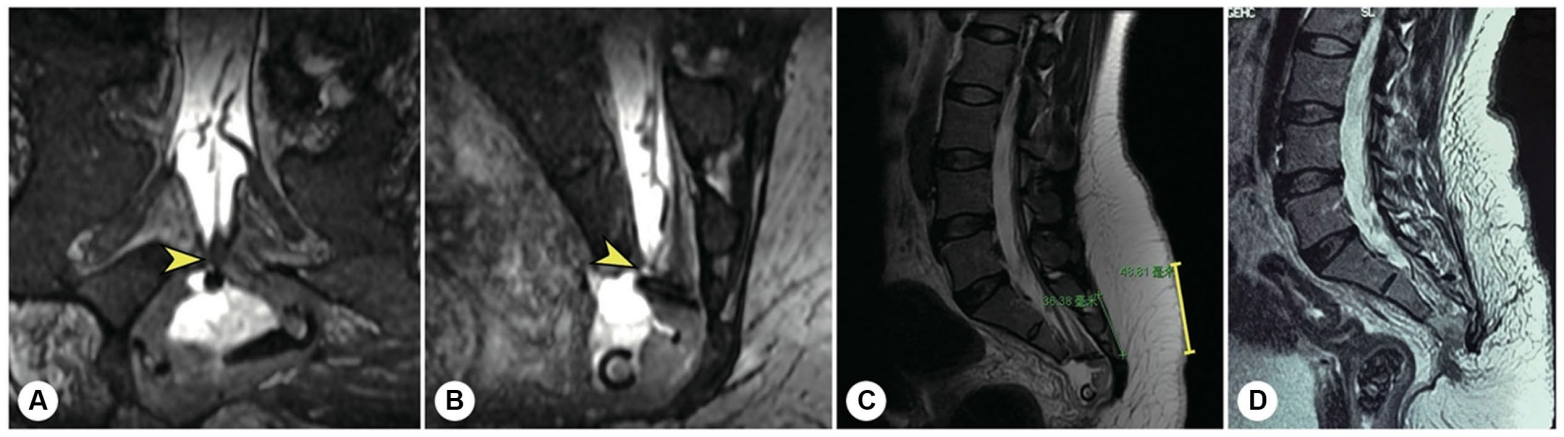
The other representative patient had both sacroanterior and sacral cysts. A 45 years-old female presenting with abdominal pain was found to have a pelvic mass on CT at the local hospital. The intrapelvic part of the cyst was resected under laparoscopy. (A) After referral to our institute, coronal reconstructed MR image showed a narrow-neck leakage orificium (yellow arrowhead) between the end of the dural sac and the sacral canal cyst, and the filum terminale was thickened. (B) Sagittal reconstructed MR image showing the leakage orificium (yellow arrowhead) at the end of the dural sac connected to the sacral cyst and the residual sac. (C) A 4 cm precise incision was made (yellow line segment). (D) After the leakage orificium was ligated and the tethered spinal cord was released, a follow-up MRI showed that the cyst had completely vanished.
